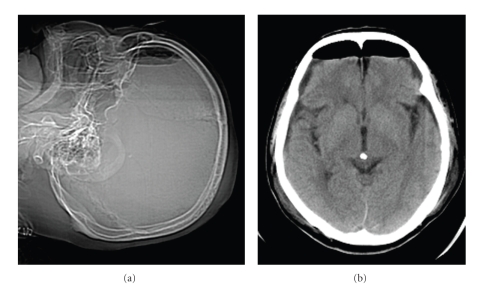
Head image ((a): Plain radiograph, (b): CT). (a) Plain radiograph demonstrates significant intracranial air. (b) CT reveals massive pneumocephalus involving the subarachnoid spaces. CT: Computed tomography.
